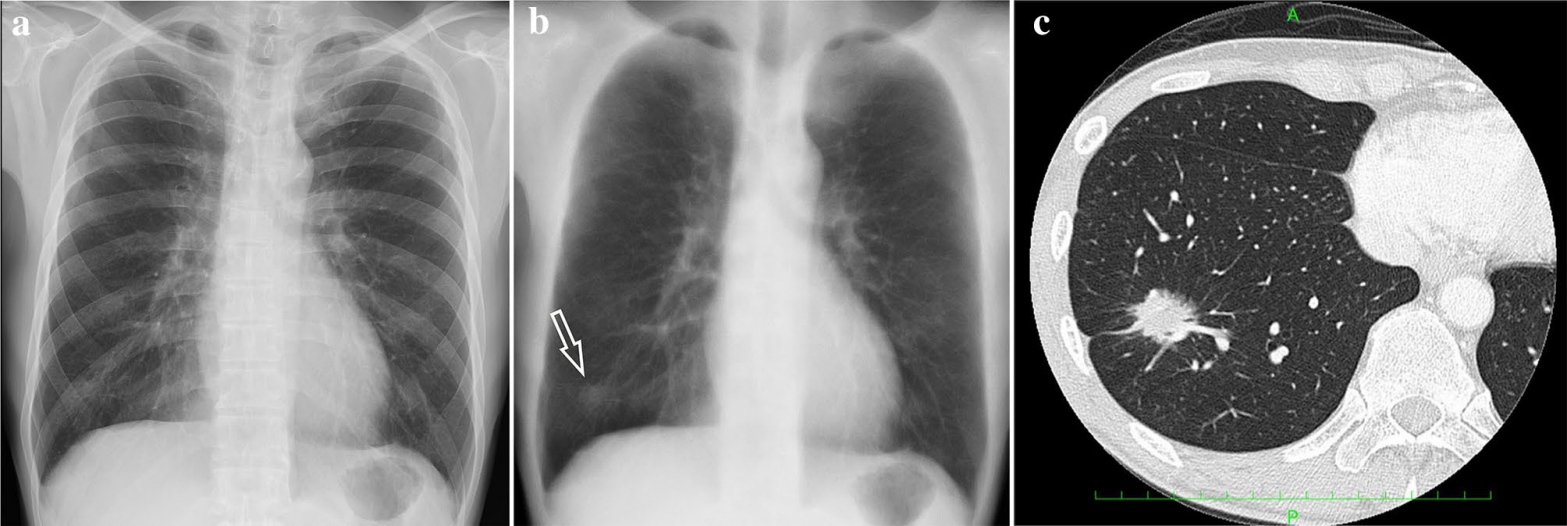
Male, 60 s, adenocarcinoma, 30 mm. a No abnormal findings were observed on conventional radiography. b The soft tissue image showed a nodular shadow in the right lower lung field (arrow). c CT showed a solid nodular shadow with GGA in the right lower lobe
